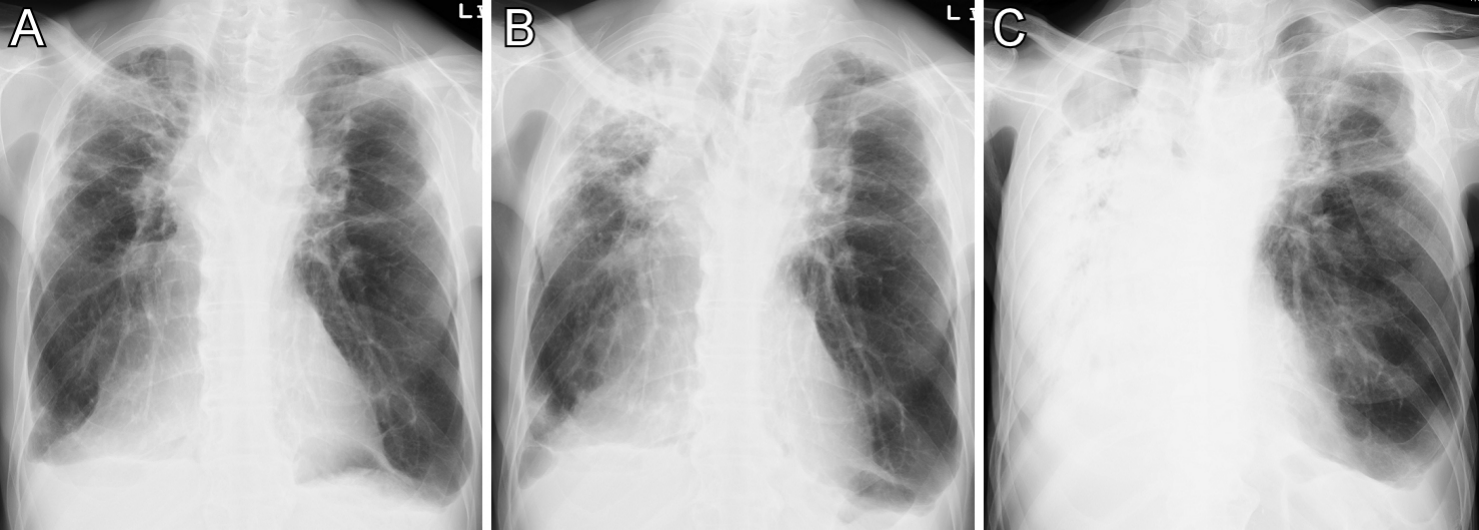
Chest X-ray on initial consultation (A), on admission for pneumonia in the right upper lobe a year later (B), and at the terminal stage with right-sided massive pneumonia a year and three months after the initial consultation (C).
